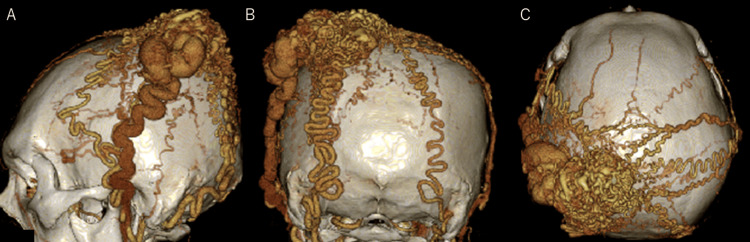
Preoperative 3DCTA (A: left lateral view, B: posterior view, C: superior view) 3DCTA demonstrates a nidus-like vascular cluster in the left temporal region. The feeding arteries are the bilateral superficial temporal arteries and the occipital artery, while the draining vein is the left superficial temporal vein. 3DCTA: three-dimensional computed tomography angiography
